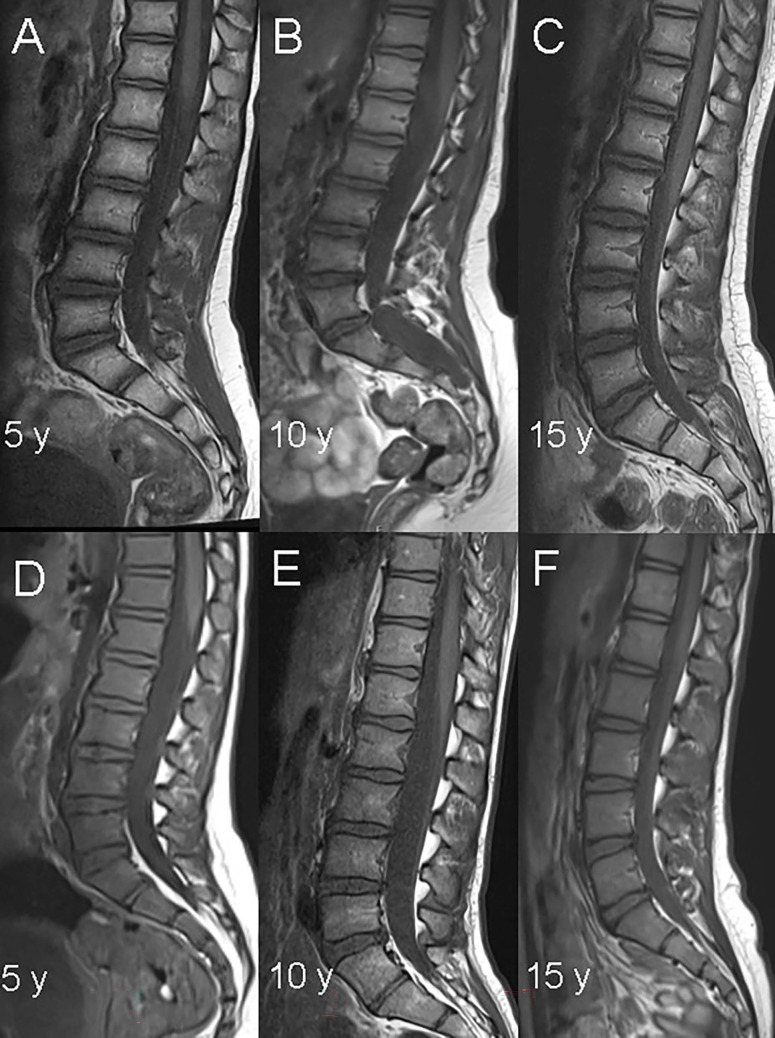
Representative sagittal T1-weighted MR images of patients with low bone mineral density at ages 5, 10, and 15 years (A–C, respectively) and age-matched controls (D–F, respectively)
